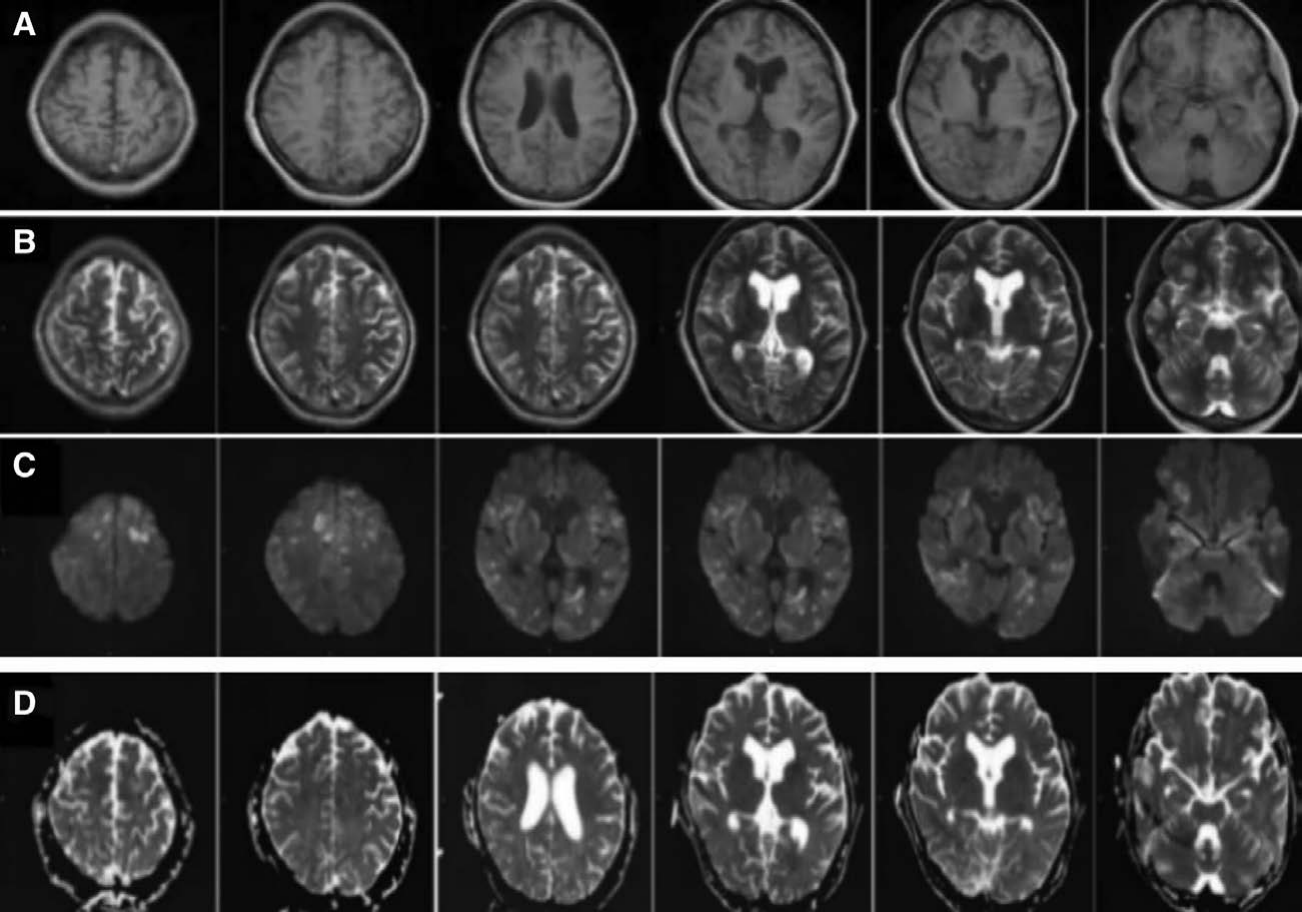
The cortex and white matter in both cerebral hemispheres had long T1 and T2 signals and a high signal in the FLAIR image. The lesion in DWI with high signal intensity and ADC with low signal intensity.
